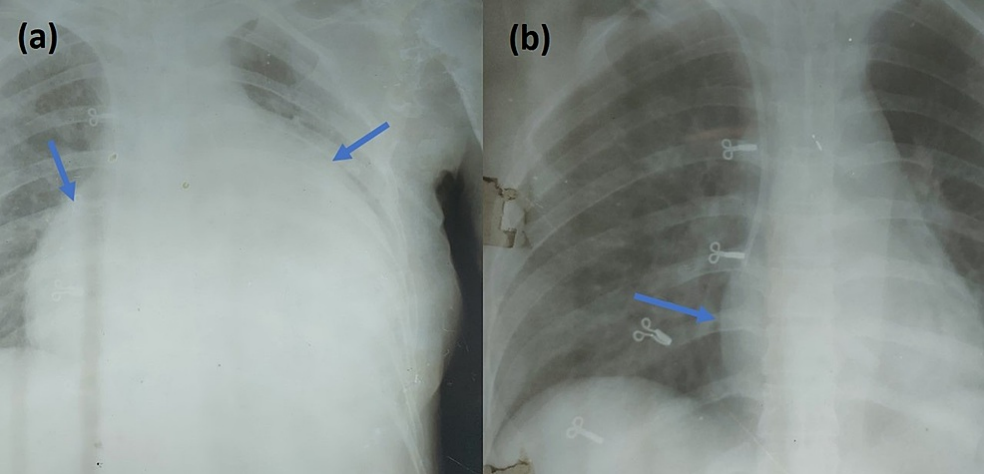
Chest X-ray showing bilaterally enlarged cardiac silhouette (a) and cardiac silhouette after pericardiocentesis (b).
